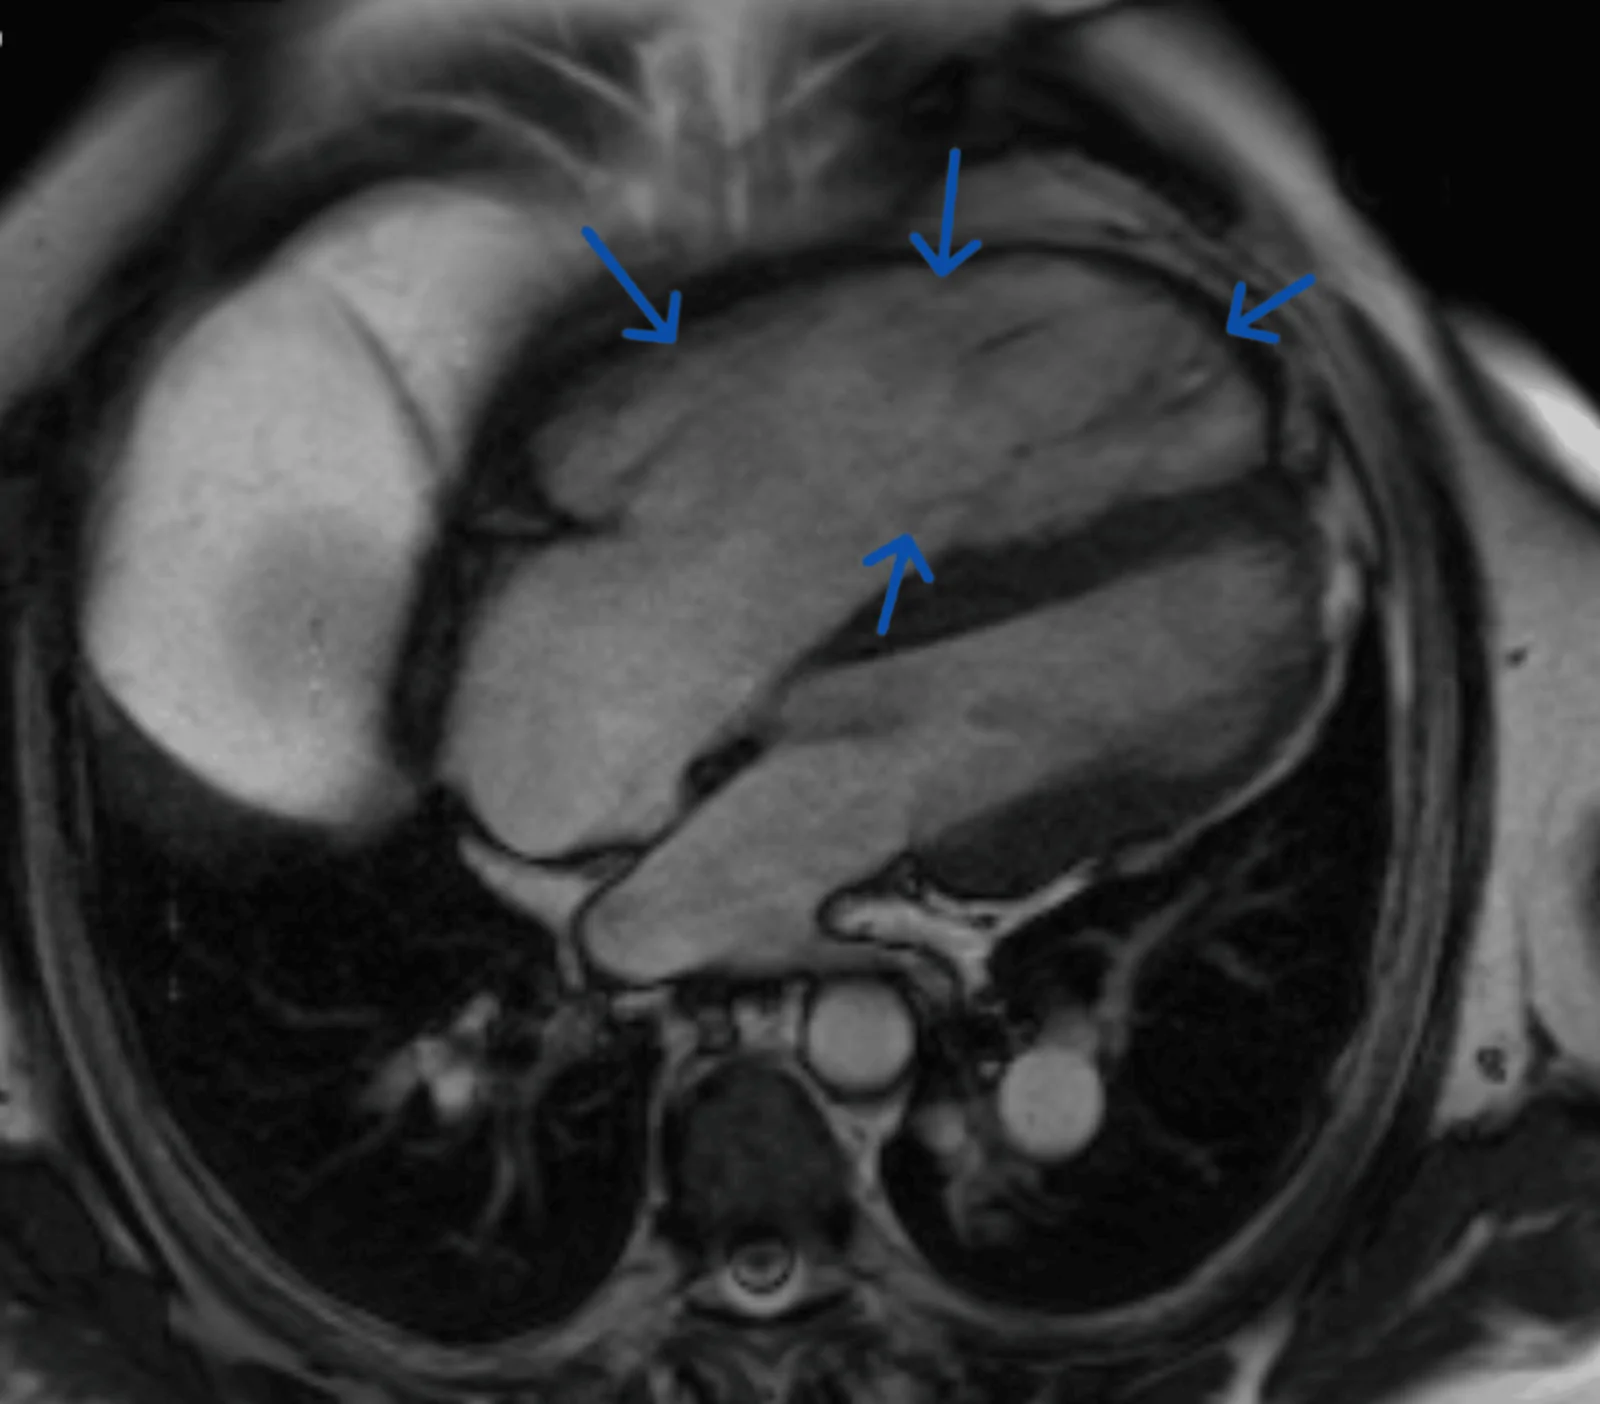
Cardiac MRI scan showing a significantly dilated right ventricle (blue arrows)
